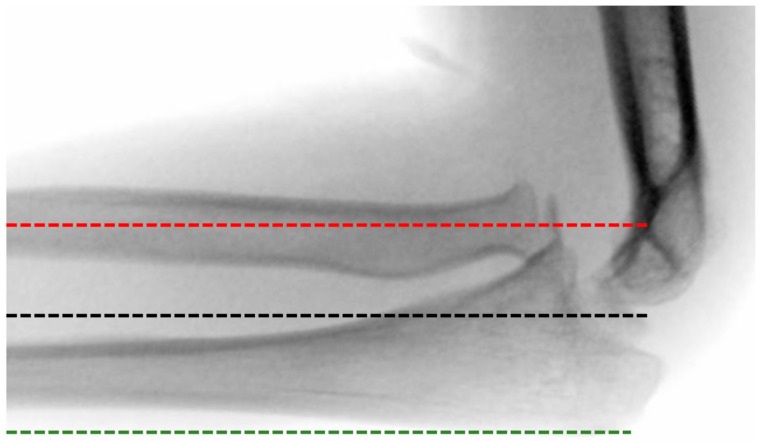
Lateral radiograph of the elbow joint in an 8-year-old girl with a missed Monteggia fracture-dislocation. In preoperative planning, the Støeren line (red line) clearly deviates from the normal radiocapitellar line (black line) and does not intersect the humeral capitulum. Additionally, the ulna already shows plastic deformation, reflected in the ulnar bow sign (green line).
